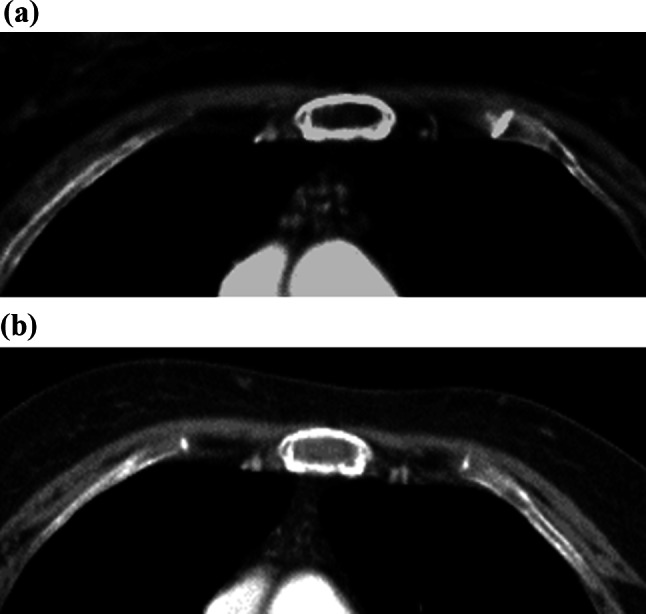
a, b A woman in her 70s receiving G-CSF for chemotherapy-induced neutropenia. CT demonstrates that the fatty marrow in the sternum (a, baseline) has changed to soft-tissue–like attenuation (b, post-G-CSF), representing hematopoietic marrow reconversion from yellow to red marrow. Caution is required to avoid misinterpretation as metastatic disease
